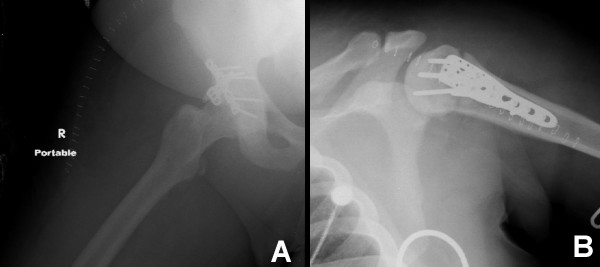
Post fixation radiographs of acetabular fracture dislocation and proximal humerus fracture.
